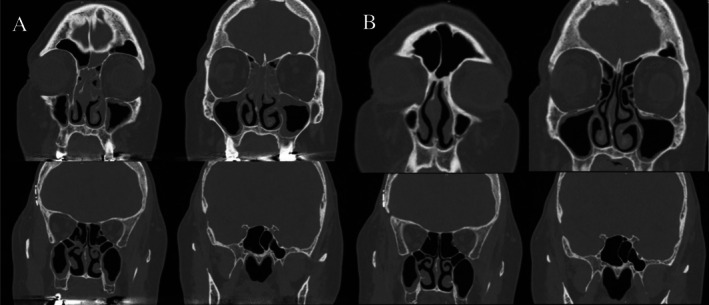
(A‐B): Patient 2 CT Imaging of sinuses before (A) and after (B) completing 6 weeks of steroid therapy for immune‐mediated sinusitis.
